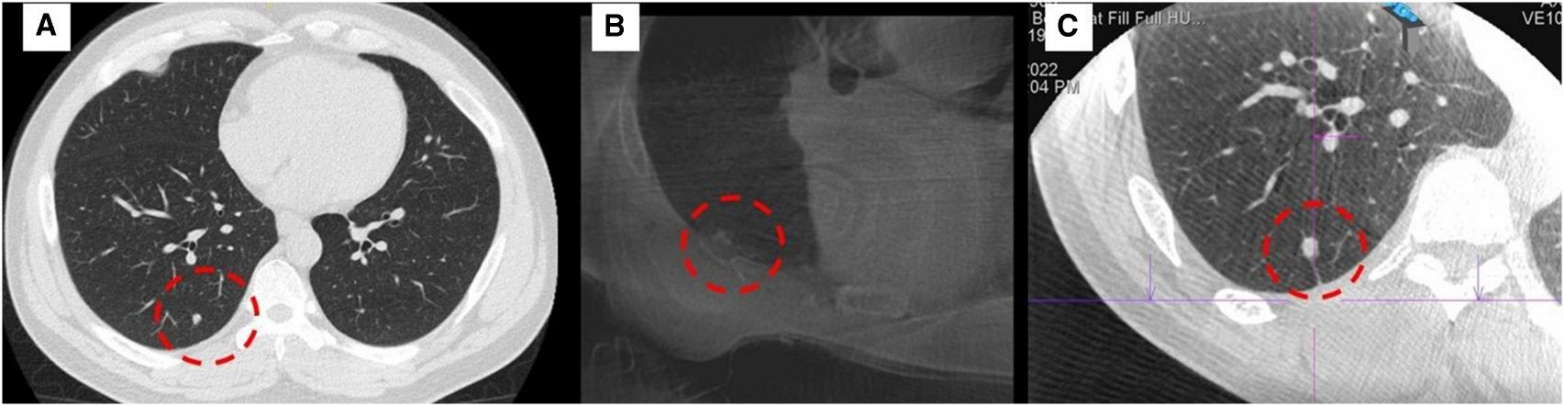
Imaging Comparison in the Failed Prone Localization Case. The red-dotted circle indicates the location of lesions. (A) Preoperative CT image demonstrating the pulmonary lesion. (B) Intraoperative 3D C-arm image acquired with the patient in prone position on a foam-and-gel surgical pad. The lesion could not be clearly identified due to compromised image quality, likely caused by the pad attenuating X-ray penetration. (C) Cone-beam CT (CBCT) image obtained in the hybrid operating room (HOR) after repositioning, which successfully delineated the target lesion and allowed for accurate localization
